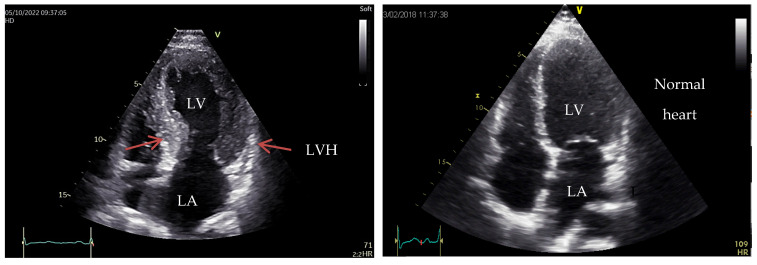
Two-dimensional echocardiography, apical four-chamber view showing important LV hypertrophy (LVH) and dilation of LA. Versus normal heart to the right side of the image.
